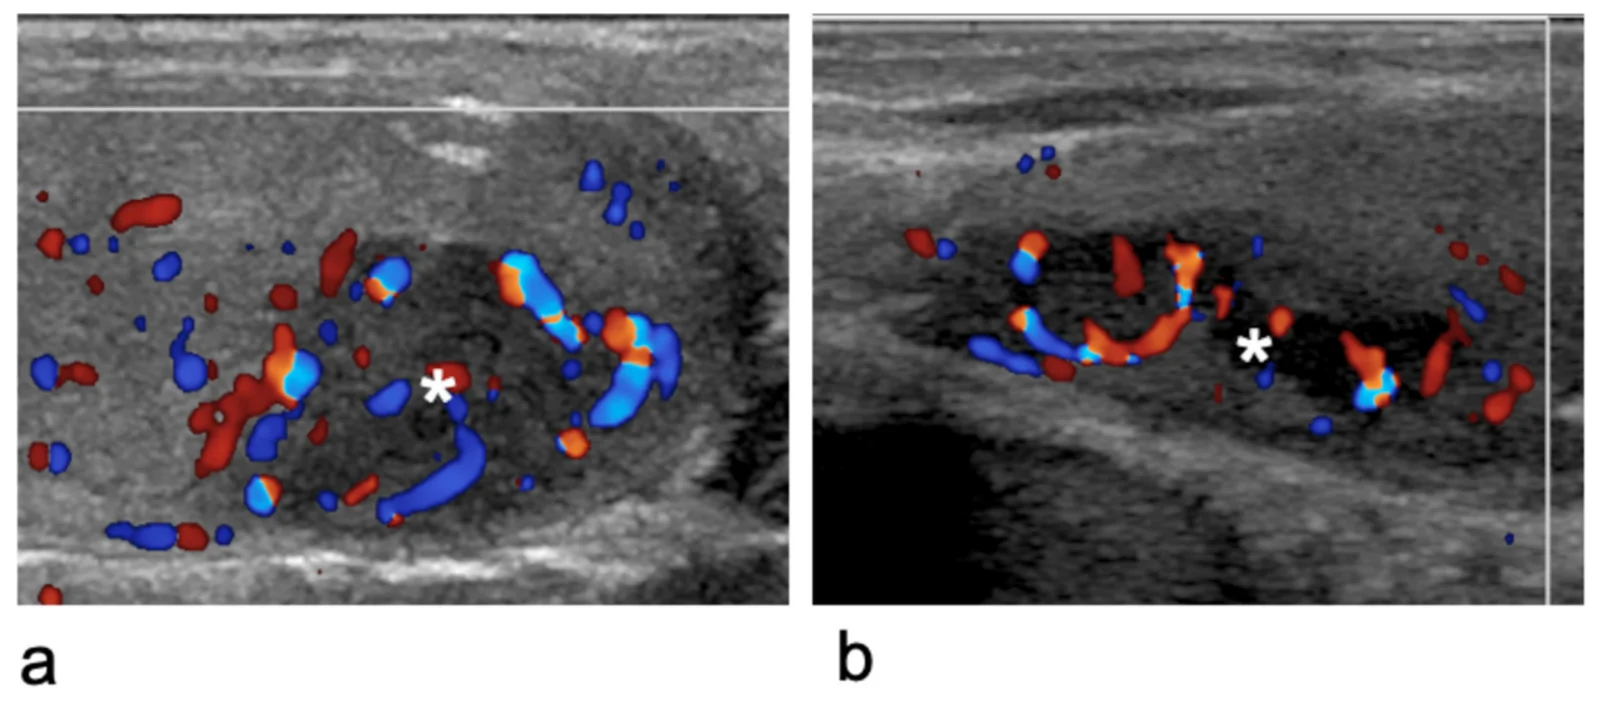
A 46-year-old man was referred for ultrasound for infertility. (a,b) Right (a) and left (b) non-palpable hypoechoic testicular lesions (asterisks) with hypervascularity were detected on ultrasound. Tumour markers, ACTH, 17-OHP and androstenedione levels were normal, making a diagnosis of testicular adrenal rest tumours unlikely. At surgery, the lesions proved to be bilateral pure synchronous seminomas.
